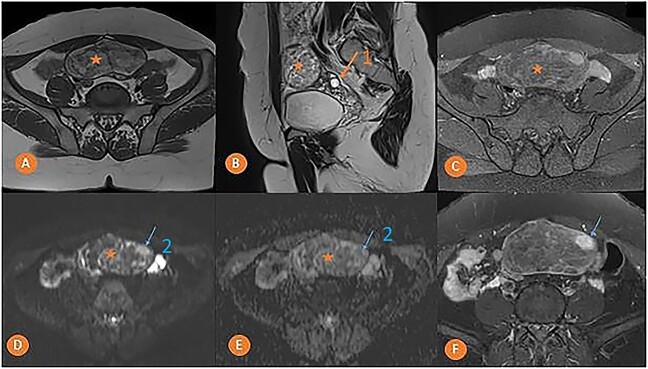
Pelvic MRI in axial T1 (A), sagittal T2 (B), axial T1 FS (C), axial DWI (D) with ADC mapping (E), and axial T1 injected (F) slices showing a median pelvic mass (star) lateralized on the left in contact with the homolateral ovary (arrow 1) with heterogeneous signal, mostly hyper intense in T1 and T2. It fades after removal of the fat and is not diffusion-restrictive except for a small nodular area on the left anterolateral side (arrow 2) which takes on strong contrast after injection of the gadolinium chelate.
